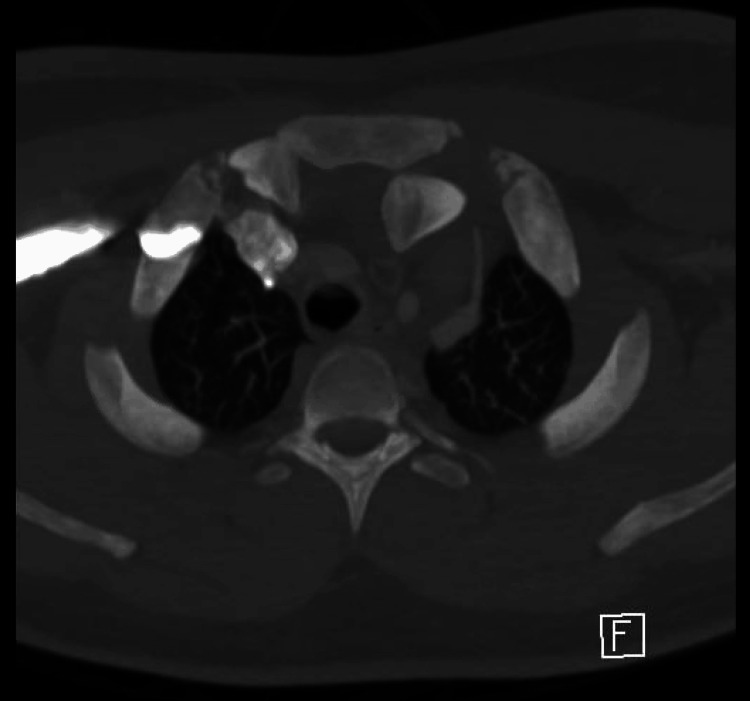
Axial chest CT angiography showing posterior dislocation of the left sternoclavicular joint.
